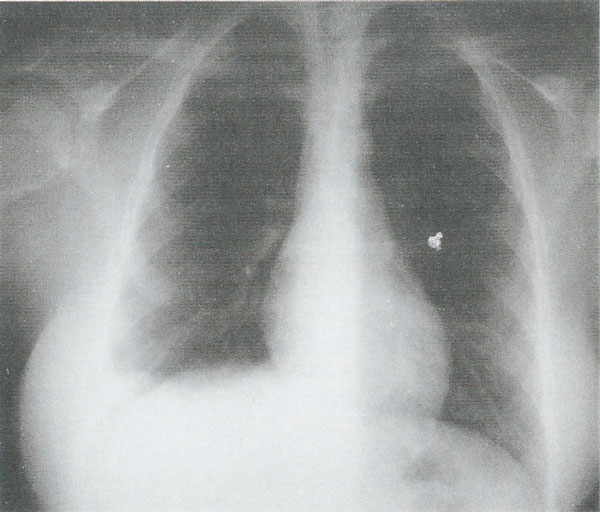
Chest X-ray of the patient showing right-sided heamothorax.
